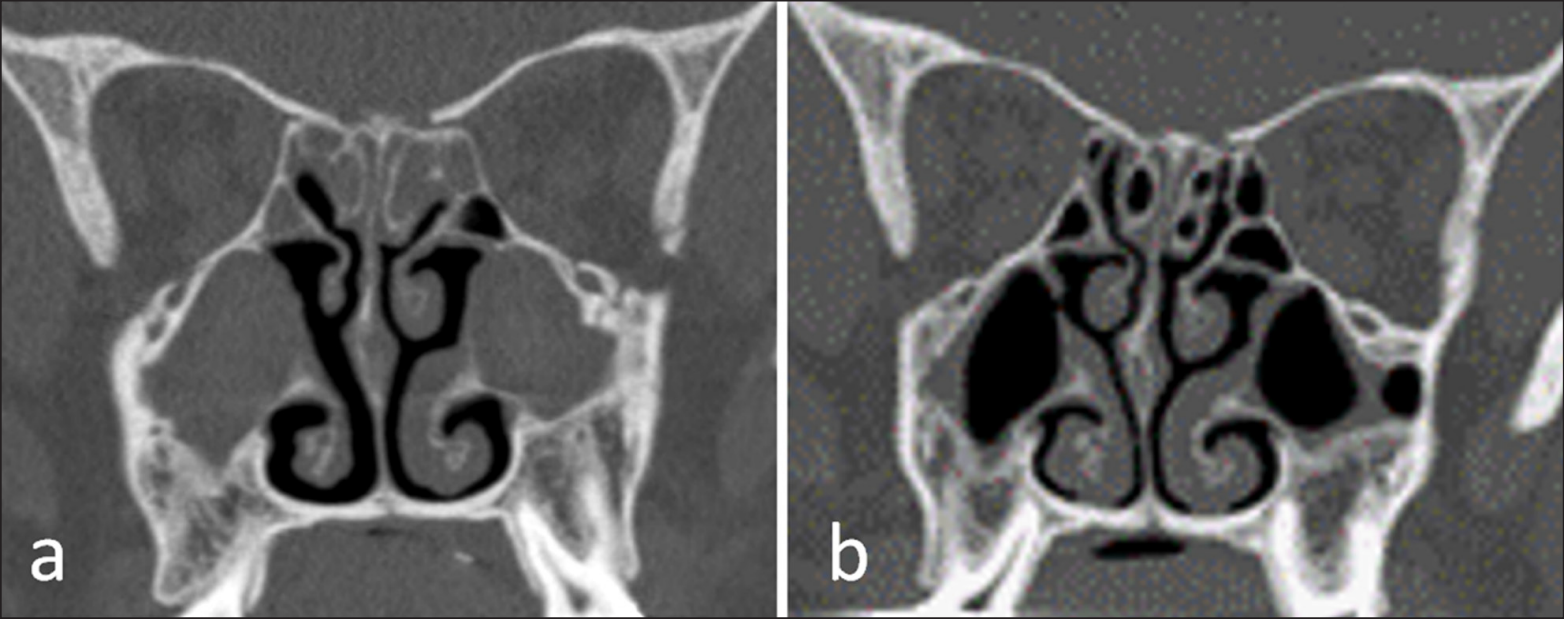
Coronal sinus computed tomography images from a cystic fibrosis patient with chronic rhinosinusitis before (a) and after (b) one year of modulating therapy.
